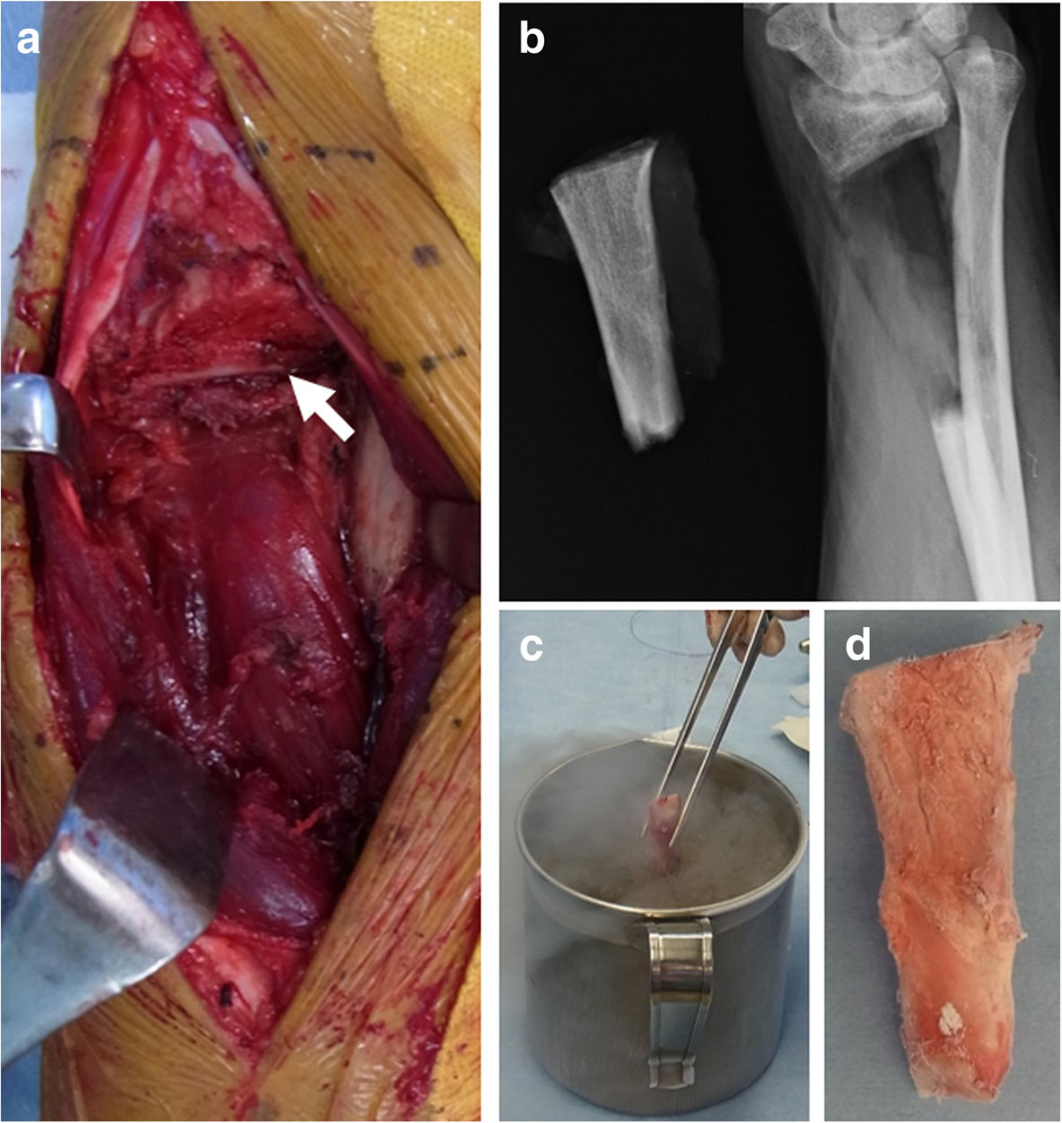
Intraoperative photos (a) The dorsal approach at the flexible side of the radiocarpal joint. The tumor bearing bone was resected with a biopsy tract, pronator quadratus muscle, and intraosseous membrane. The main extensor and flexor tendons were preserved. b Intraoperative radiograph of the tumor bearing bone resected along the planned osteotomy line. c The tumor bearing bone was frozen in liquid nitrogen. d After freezing
